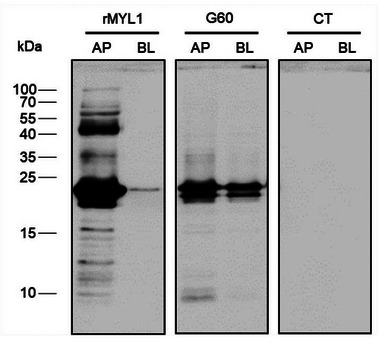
rMYL1 is transported intact through a monolayer of Caco‐2 cells. Anti‐rMYL1 immunoblot of the medium applied to the apical (AP) side and collected from the basolateral side (BL) of a Caco‐2 cell monolayer cultured on permeable supports. Cells were incubated with undigested rMYL1, with rMYL1 digested by pepsin for 60 min (G60) or with medium (CT) only. Molecular weights (kDa) are indicated in the left margin.
